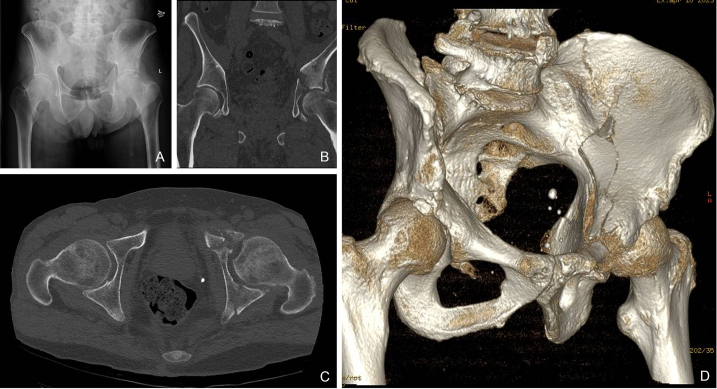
Preoperative plan images. (A) Antero-posterior X-ray, (B) (C) CT scans and (D) 3D reconstruction of the hip of case 4, who fractured its left acetabulum on both columns and injured the femoral head, after an accidental fall.
